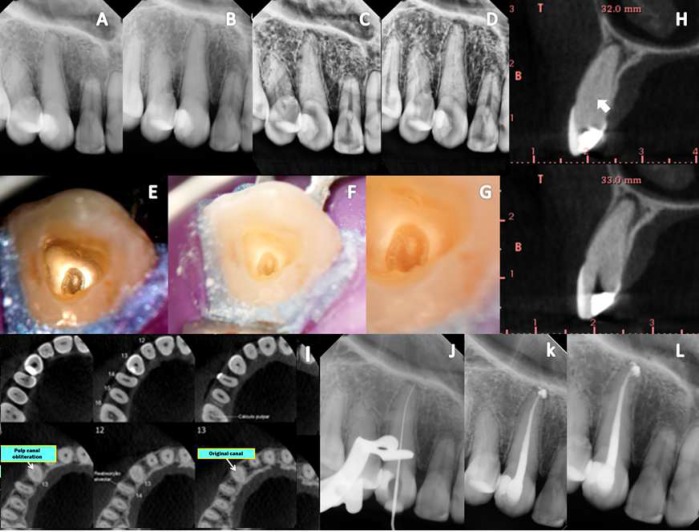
Case 3: *A, B)* DR was performed at multiple angles before the cavity was accessed; *C, D)* Evaluation of DR using the periodontics filter digital system XDR®; *E, F, G)* Identification of a yellowish area, which was suggestive of the original canal, via DOM; *H, I)* CBCT sagittal and axial slices: obliteration of the canal in the cervical third and the presence of light in the middle and apical thirds (ARROWS); *J)* DR: canal identification; *K)* Final DR: canal fillings; *L)* Follow-up imaging after 1 year of treatment*
